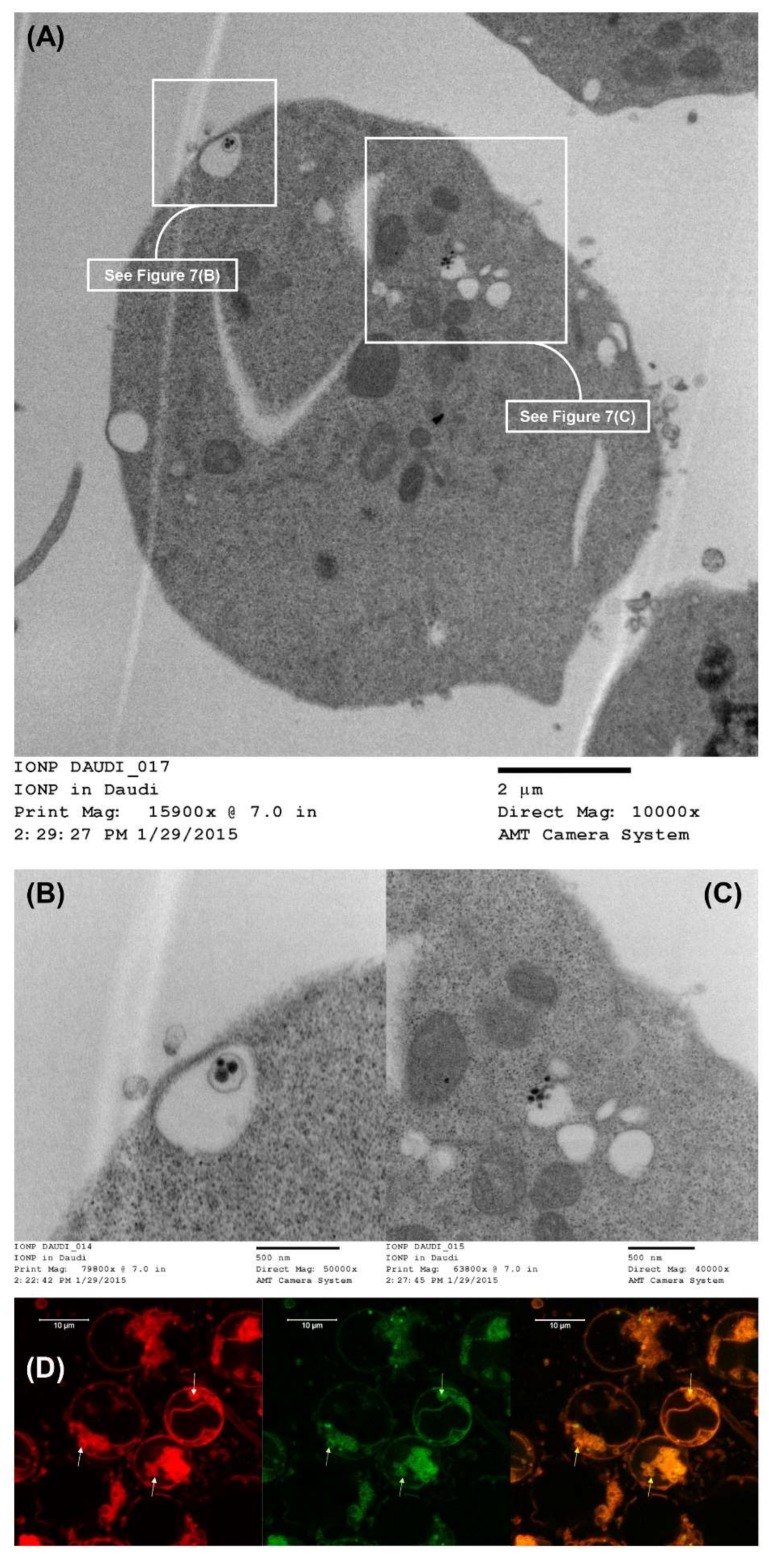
(A) TEM image of LCCTLA intracellular localization in Daudi cells; (B) Enlarged from Figure 7A showing an MLV of MLCCTLA inside an endosomal structure; (C) Enlarged from Figure 7A showing SUV’s of MLCCTLA inside an endosomal structure; and (D) CLSM image of intracellular localization of LCCTLA in endolysosomes.
